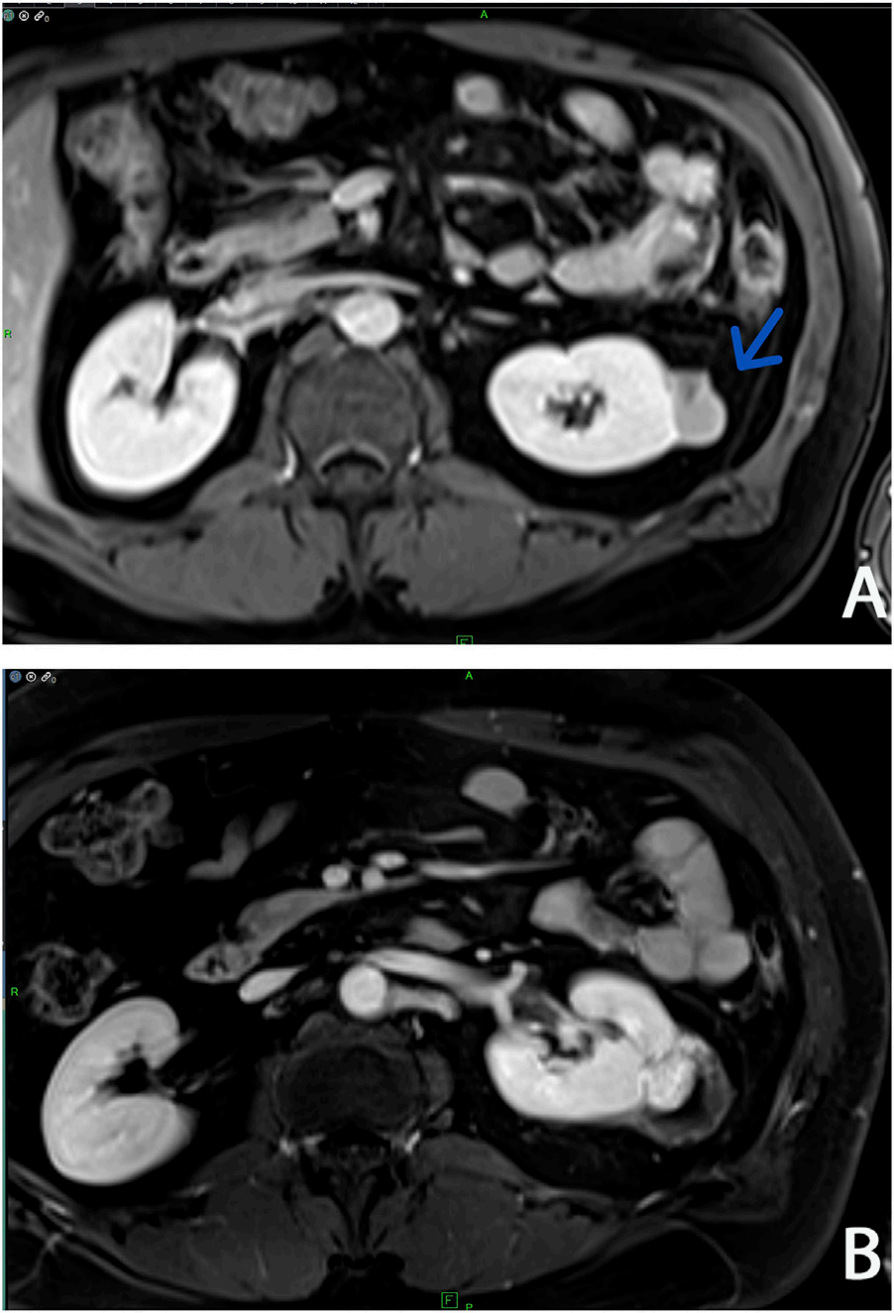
Abdominal contrast-enhanced T1-weighted MRI imagings at two different times: (A) Arrow shows a localized complex cystic lesion (Bosniak III, cyst) on the left kidney (B) Three years later an increasing cystic lesion with hyperenhanced solid component (Bosniak IV, cystic tumor) was detected on the same side.
